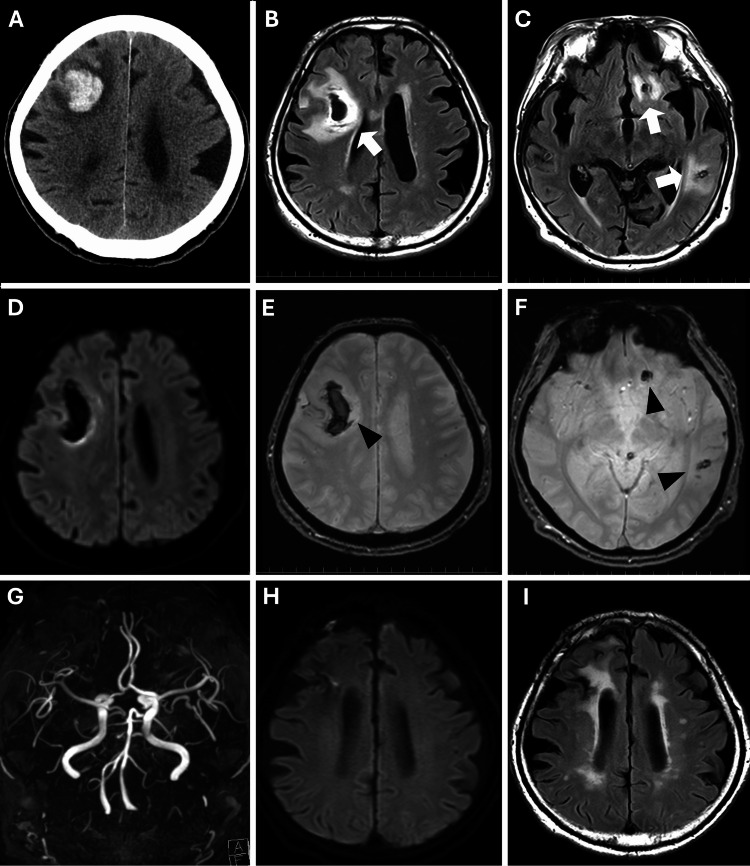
Neuroimaging findings (A) Head CT showing a large cortical-subcortical hemorrhage in the right frontal lobe. (B, C) FLAIR MRI highlighting edema and hyperintense signals surrounding the hemorrhagic lesion in the bilateral frontal lobes and the left temporal lobe (arrows). (D) DWI MRI showing low intense signal in the right frontal lobe. (E, F) T2-star MRI demonstrating susceptibility changes consistent with hemorrhages (arrowheads). (G) MRA revealing no significant abnormalities in the major cerebral arteries. (H, I) Follow-up MRI two months after treatment showed lesion reduction on both DWI and FLAIR. FLAIR: fluid-attenuated inversion recovery; DWI: diffusion-weighted imaging; MRA: magnetic resonance angiography
